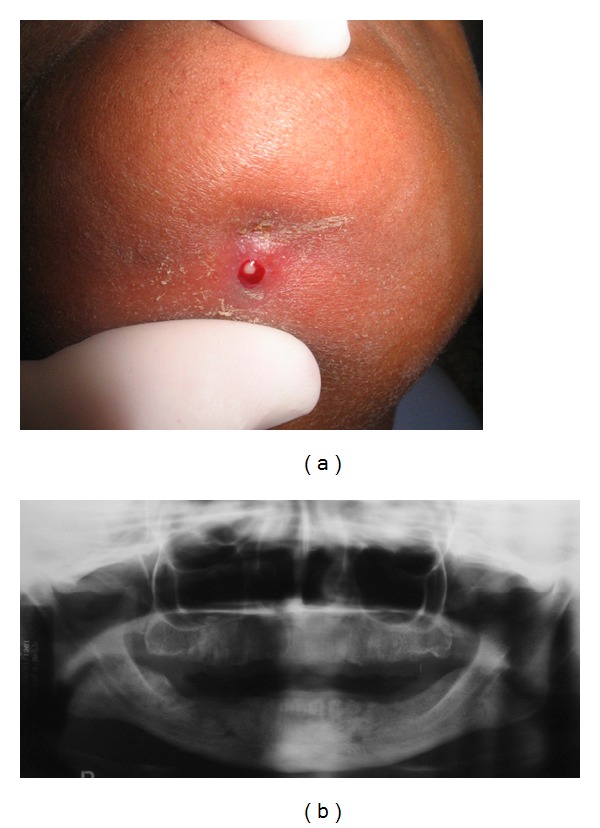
((a) and (b)) Clinical aspect of BRONJ showing purulent drainage in the submental region and absence of radiographic changes.
